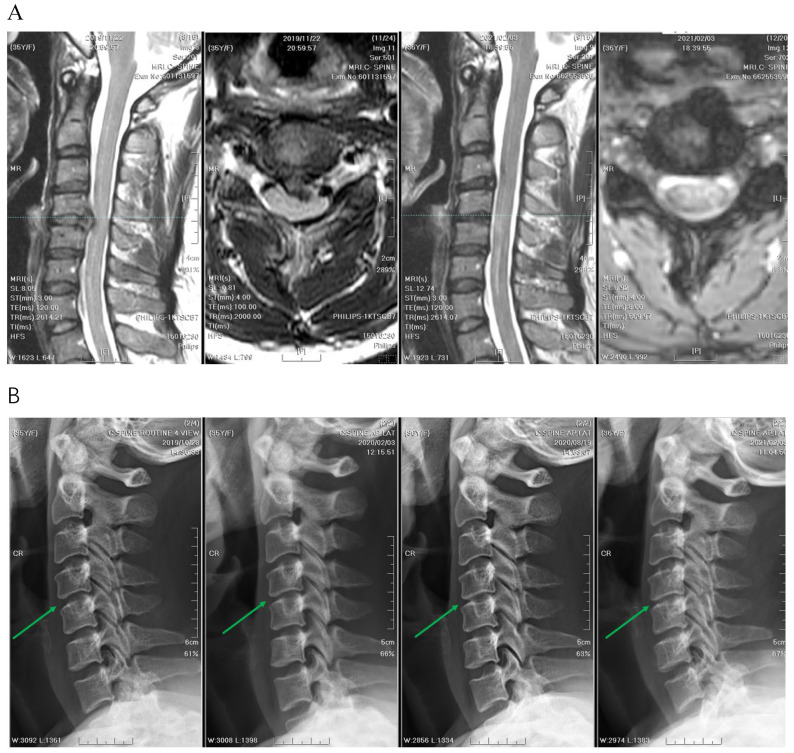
(A) The intervertebral disc height of C4–C5 decreased significantly from 4.4 mm to 3.8 mm, before and after APECD (MRI before APECD on the left and 1 year after the surgery on the right). (B) The images from left to right are radiographs before APECD, and 3 months, 6 months, and 1 year after the surgery. Green arrows point to the C4–C5 disc space. The reduction of disc height of C4–C5 was sufficient to be observed by the naked eye.
